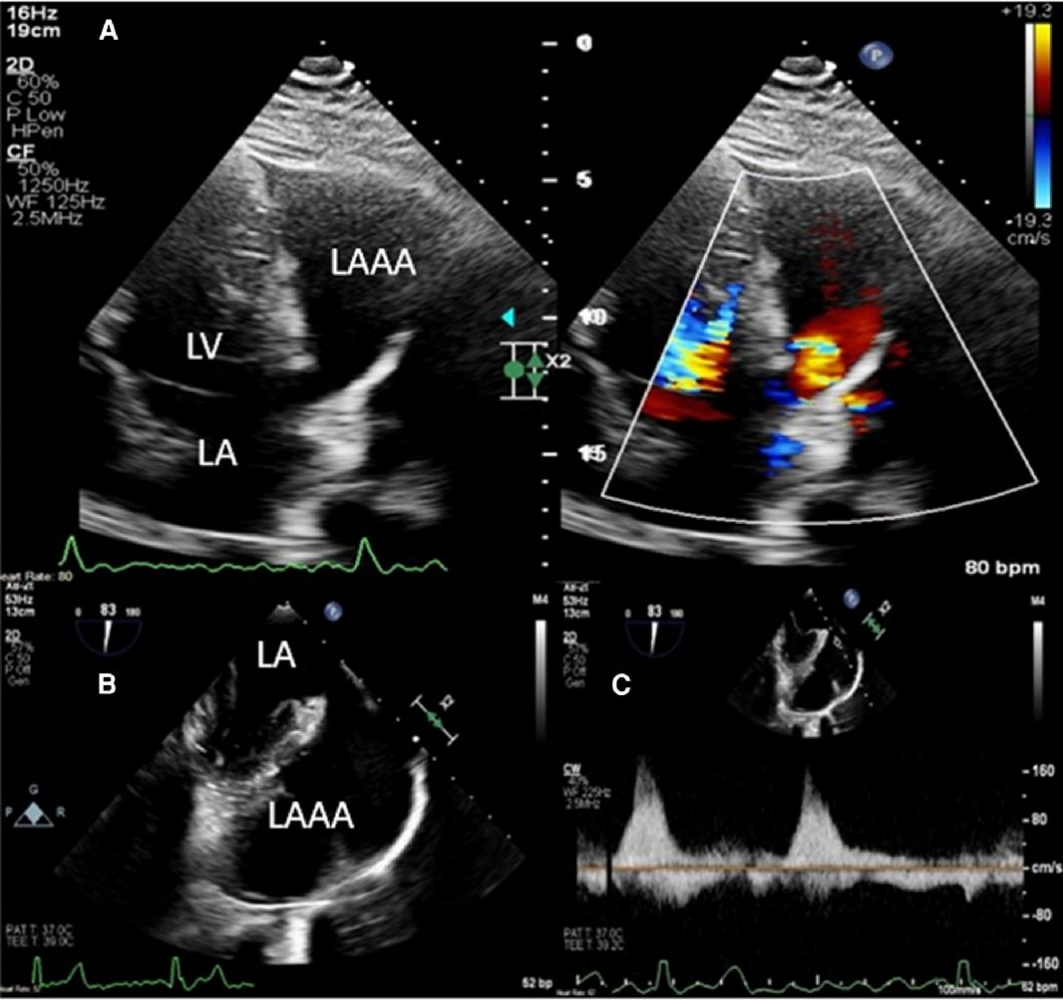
(*A*) Transthoracic echocardiography during pre-operative assessment in slight off-axis apical four-chamber view showing round hyperlucent structure on the lateral side of LV, which is in continuity with LA as documented with colour Doppler signals. (*B*) Intraoperative TOE at mid-oesophageal level showing LAAA with no evidence of intracavitary thrombus or spontaneous echo contrast. (*C*) Continuous wave Doppler at the level of LAAA inlet documenting normal emptying velocity of 160 cm/s.
